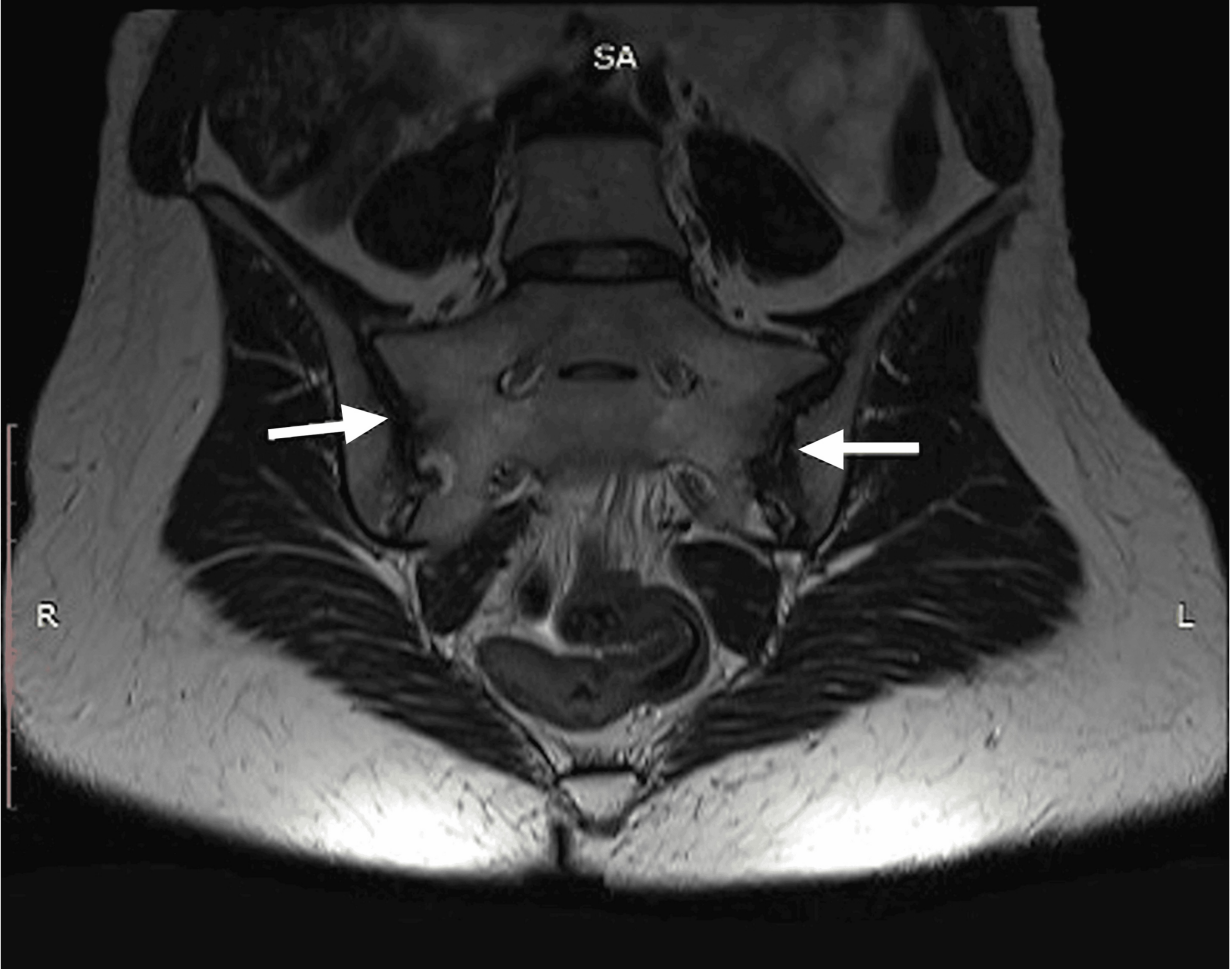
MRI of sacroiliac joints MRI conclusion: The joint space is seen throughout. Moderate bilateral joint space widening. The articular surfaces on both sides are clear and uneven with the presence of subchondral sclerosis. Some very subtle defects in the lower third of the joints are seen on both sides. Trabecular edema is present on the subchondral sections on both sides, on the right lower third of the joint, and on the left throughout. MRI: magnetic resonance imaging
